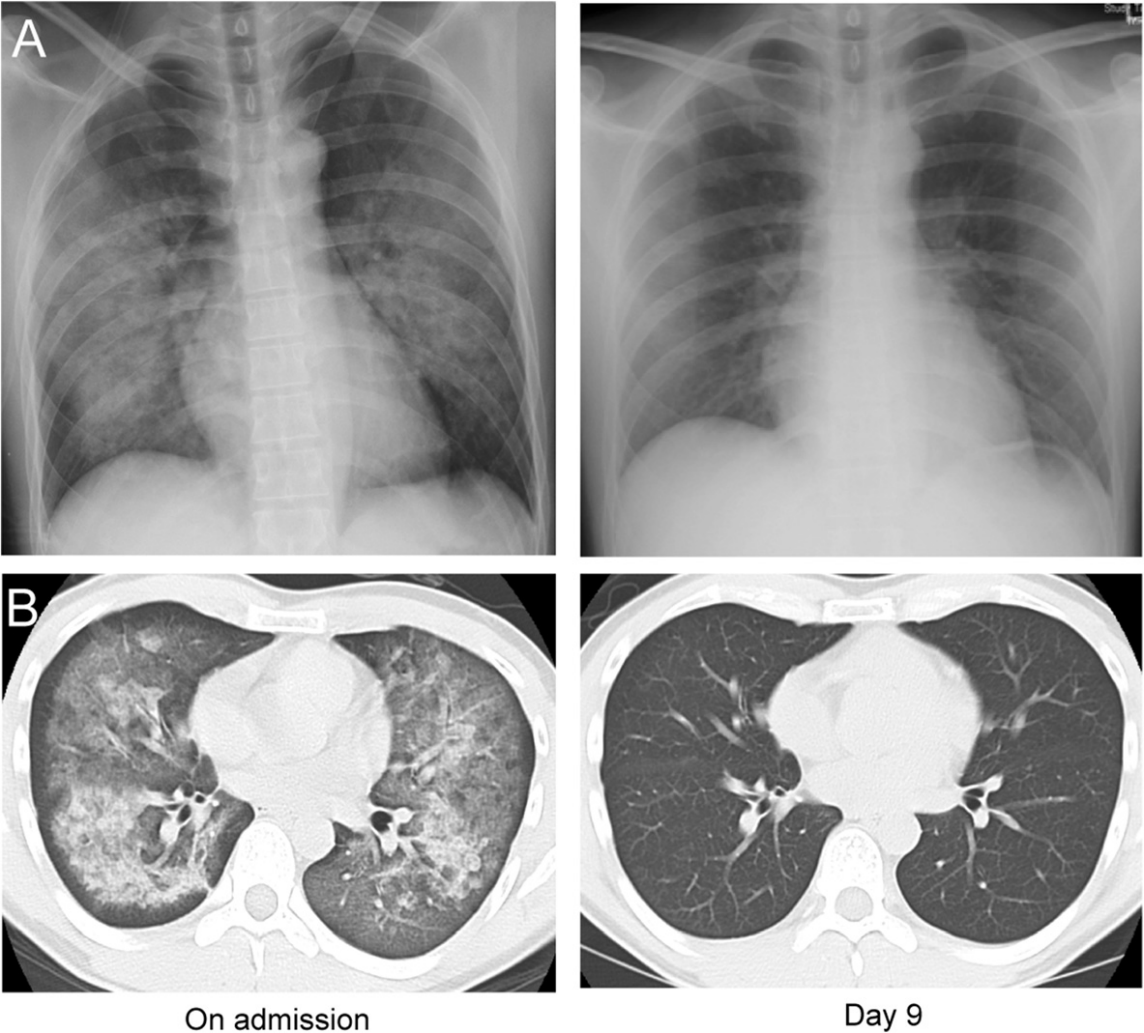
Chest X-ray and computed tomographic scan obtained upon admission and on day 9 of hospitalization. (A) Bilateral infiltration shadows were detected on admission (left), which had completely disappeared at day 9 (right). (B) On admission, bilateral patchy consolidations with ground-glass opacities and sparing of peripheral areas were found (left). On day 9 of the patient’s hospitalization, these shadows had completely disappeared (right).
